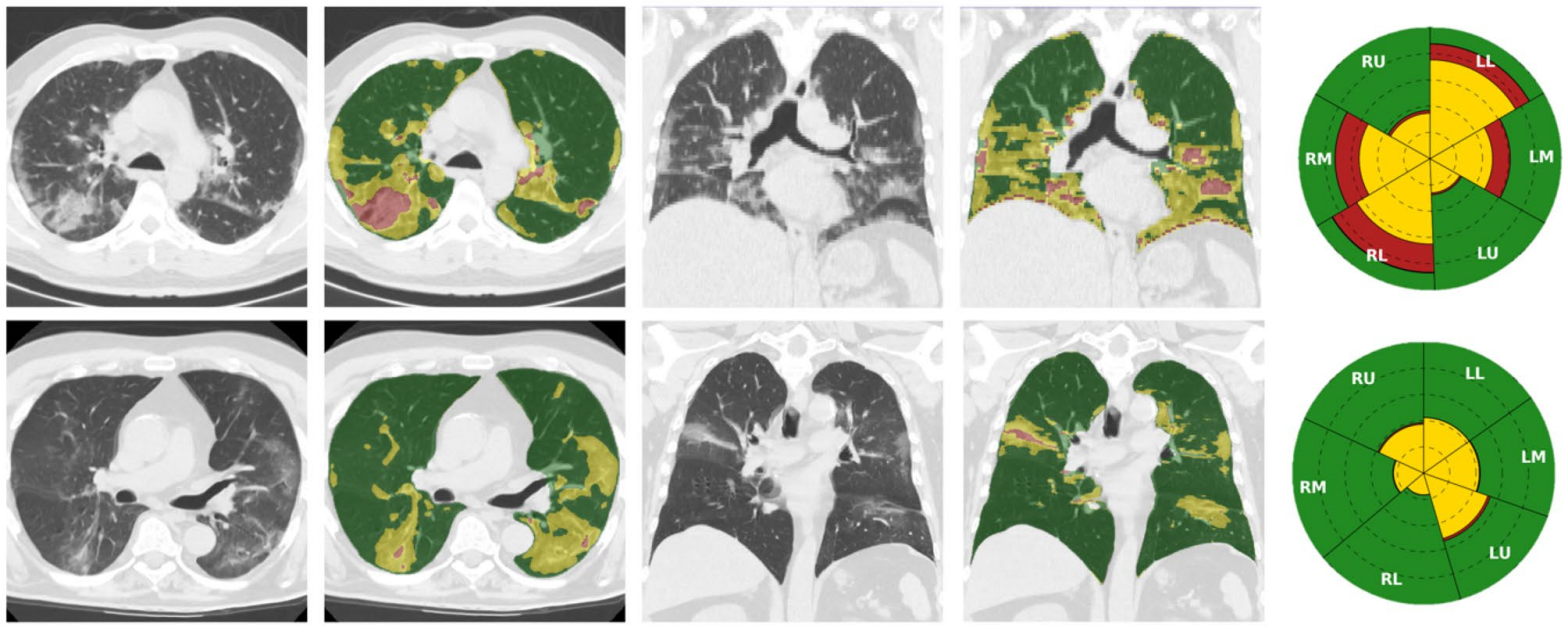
Automatic disease quantification. Nonenhanced CT axial and coronal views (first and third columns) overlaid with the automatic lesion subtyping segmentation (second and fourth columns) of two cases. Colors of the overlay correspond to normal parenchyma (green), ground glass (yellow), consolidation (reddish). First case of a 55 year old man with moderate lung involvement (upper row), and another case of a 71 year old man with mild lung involvement (lower row). The corresponding glyphs (right column) show the percentage of lung zones for each subtype shown in colors: healthy parenchyma (green), ground glass opacities (yellow) and consolidation (red). Lungs’ regions represented in the glyphs correspond to: RU- right lung upper region, RM- right lung middle region, RL- right lung lower region, LU- left lung upper region, LM- left lung middle region, LL- left lung lower region.
